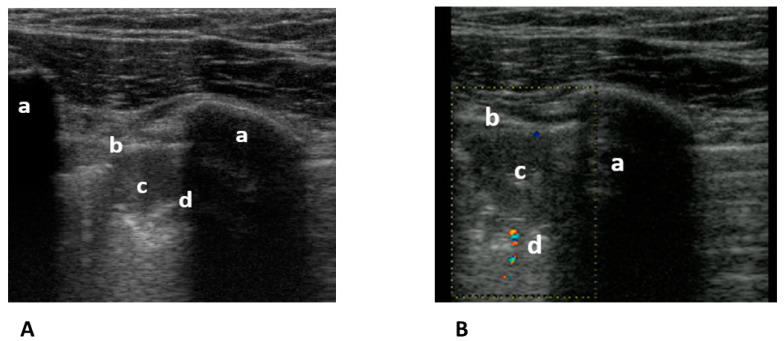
(A) Transthoracic longitudinal view: ribs (a), pleural line (b), and subpleural triangular consolidation (c) with central bronchus reflex (d). (B) Transthoracic longitudinal view: rib (a) pleural line (b), and second subpleural triangular consolidation (c) with obstructed vessel (d). Image courtesy of J. Osterwalder.
